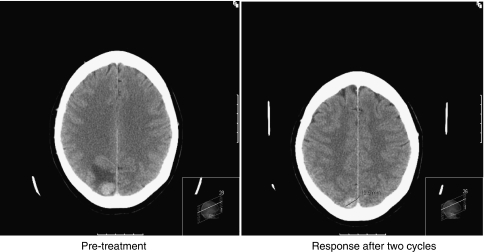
Response of CNS metastases to lapatinib and capecitabine in the absence of previous whole-brain radiotherapy. This 61-year-old woman with HER2-positive breast cancer was diagnosed in 2002. Subsequently, she received three lines of trastuzumab-containing chemotherapy for metastatic breast cancer (paclitaxel, vinorelbine and capecitabine). In July 2007, she developed headache, nausea and vomiting. CT brain revealed three metastases with associated cerebral oedema. She required only a very small dose of steroid and was entered into the LEAP study without previous local therapy (neither neurosurgery nor WBRT) and a combination of capecitabine and lapatinib was her first treatment for CNS disease. She had an excellent clinical response after two cycles, with complete resolution of her headache, nausea and intermittent vomiting, as well as radiological improvement on repeat CT scan with resolution of cerebral oedema and volume reduction of metastases.
